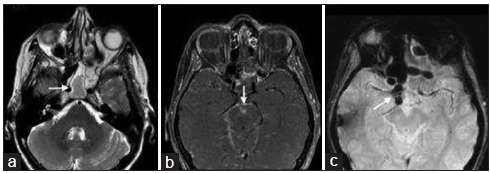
A 62-year-old woman presenting with headache and vertigo found to have meningitis, secondary to the intracranial extension from sinusitis. (a) T2-weighted axial MRI image through the brain demonstrates T2 hyperintensity within the left sphenoid and ethmoidal air cells (arrow). (b) Contrast-enhanced axial MRI image of the brain demonstrates leptomeningeal enhancement (arrow) in the interpeduncular fossa and along the cerebral peduncles, consistent with meningitis. (c) Susceptibility-weighted MRI image demonstrates round foci of susceptibility hypointensity in the parasellar region with blooming artifact (arrow), consistent with pneumocephalus.
